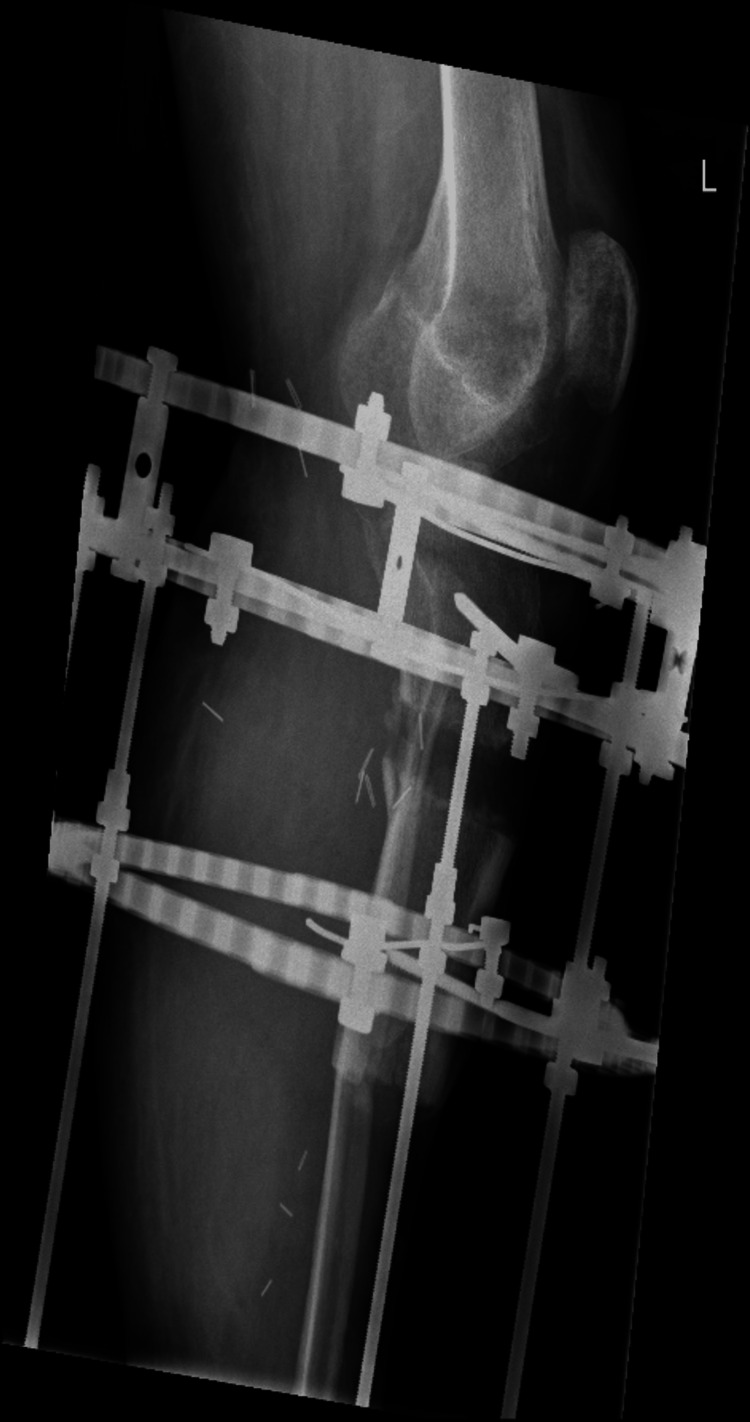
A radiograph of the left tibia showing a corticotomy and lengthening over the Ilizarov frame
